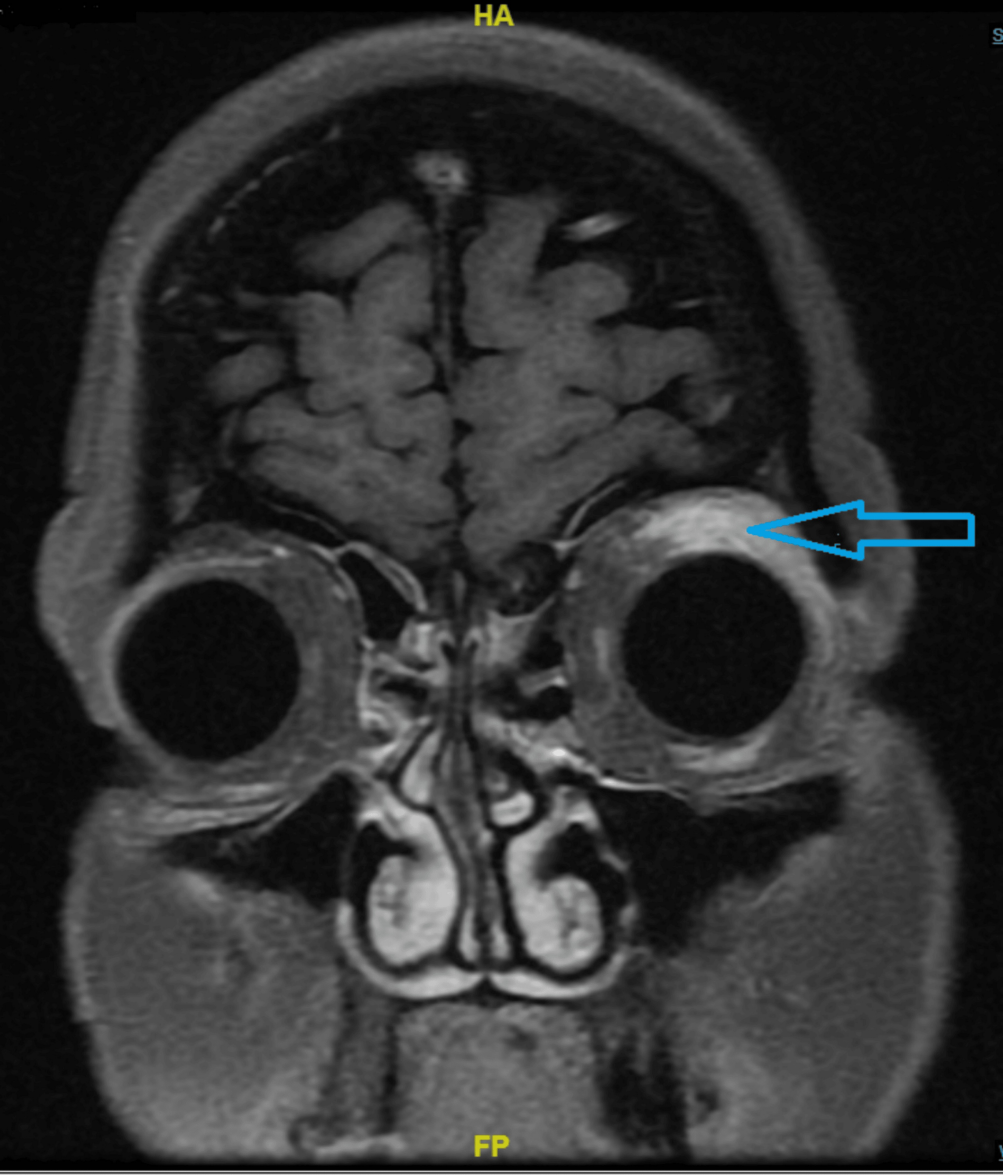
MRI scan MRI revealed T2/STIR (short tau inversion recovery) hyperintensity and focal enhancement of the left levator palpebrae superioris, superior rectus, and adjacent extraconal fat in the left superior temporal quadrant (arrow), consistent with isolated disease.
